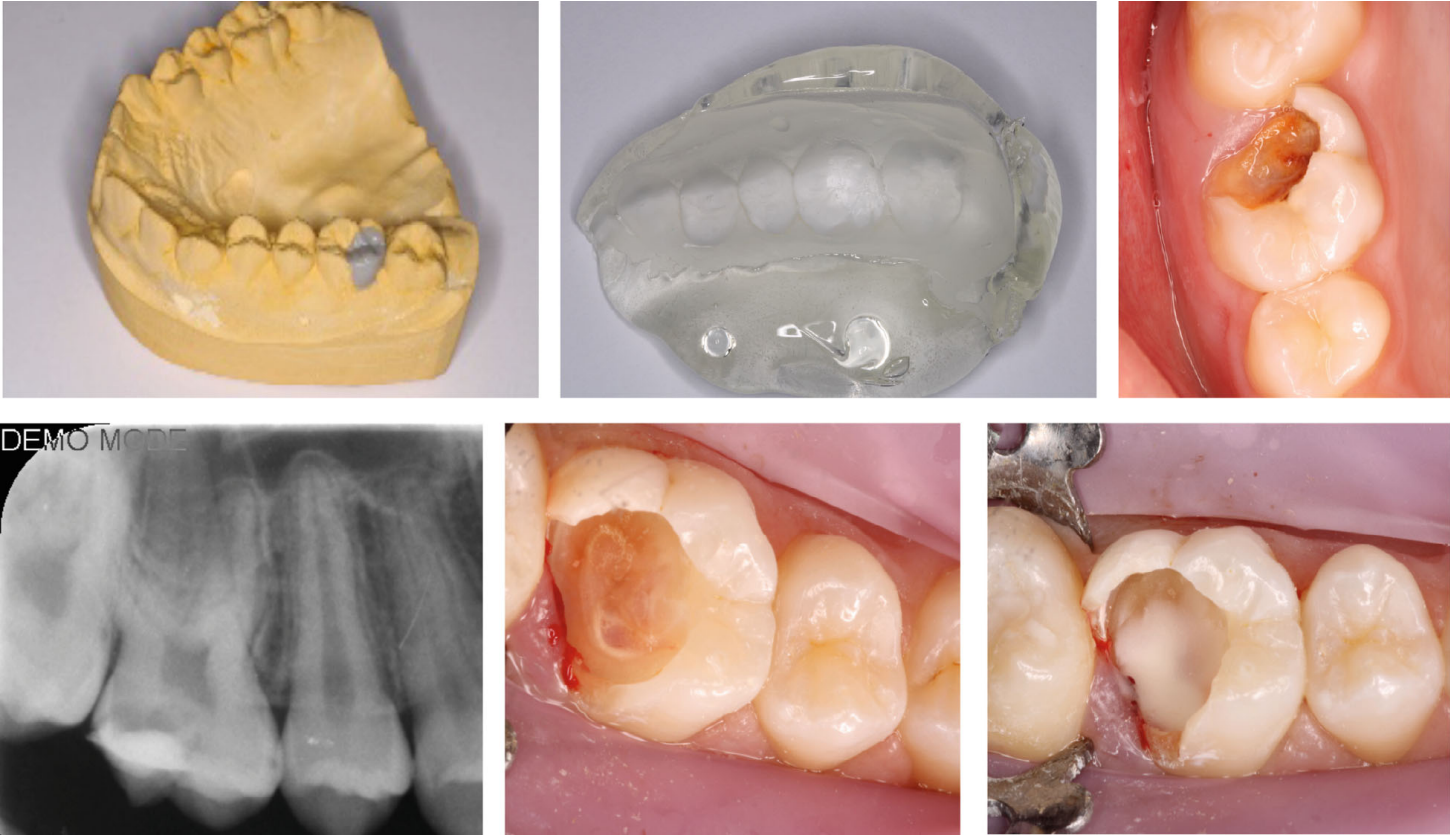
Intraoral clinical and radiographic appearance confirming an extensive occlusal carious lesion in the right upper first permanent molar involving the distobuccal cusp, in close proximity to the pulp. Preparation of a wax-up model by a specialized dental technician and fabrication of a silicone matrix.
